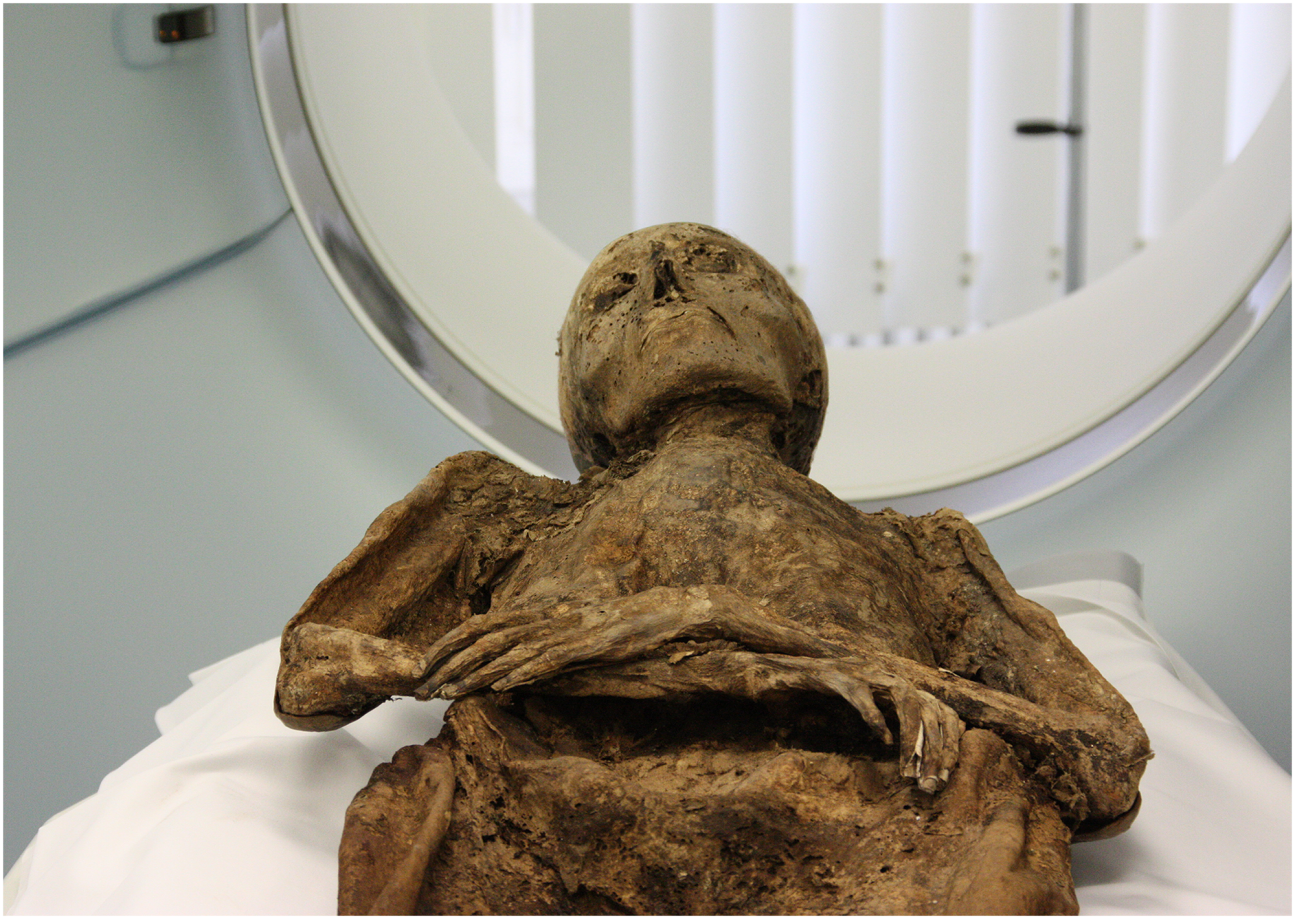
Individual A during CT scanning at Institute of Clinical Radiology and Nuclear Medicine of the University Medical Center Mannheim, Germany (photo: W. Rosendahl).
